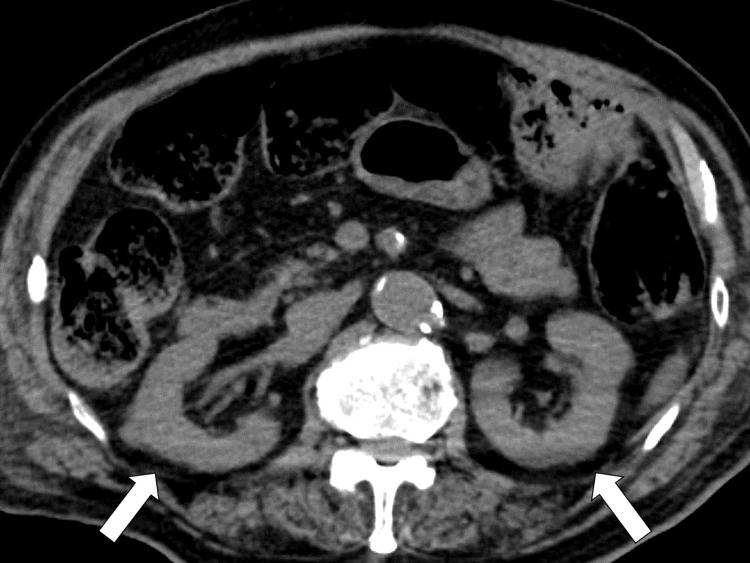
CT of the abdomen showing bilateral renal atrophy (white arrows) CT: computed tomography
